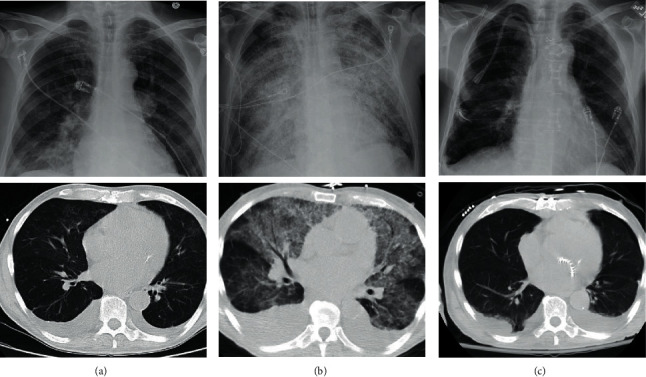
Chest imaging: chest X-ray and chest CT of progression of diffuse alveolar hemorrhage. (a) Day 5 shows right basilar opacity. (b) Day 10 shows diffuse alveolar infiltrates consistent with DAH. (c) Day 15, which was approximately five days postoperation, shows resolution of alveolar hemorrhage.
